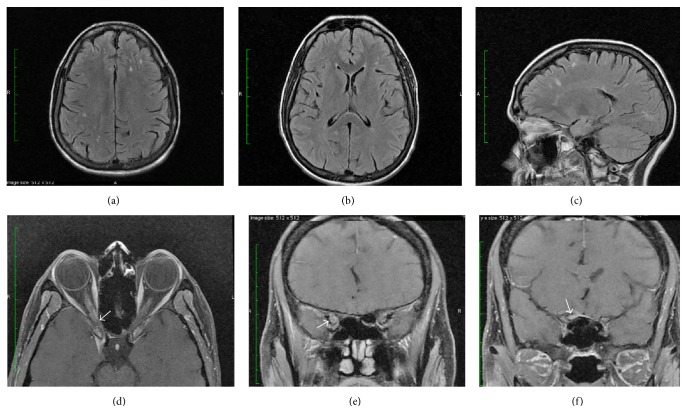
Brain MRI T2 FLAIR axial (a, b) and sagittal (c) scans made at the time of presentation showing multiple nonenhancing hyperintense lesions in the periventricular, deep, and subcortical white matter. MR contrast-enhanced axial (d) and coronal (e, f) sequences of the orbit. The arrows pointed to a small extra-axial lesion with peripheral enhancement extending into the optic canal and apex of orbits surrounding the optic nerve with a small amount of fat stranding this region.
